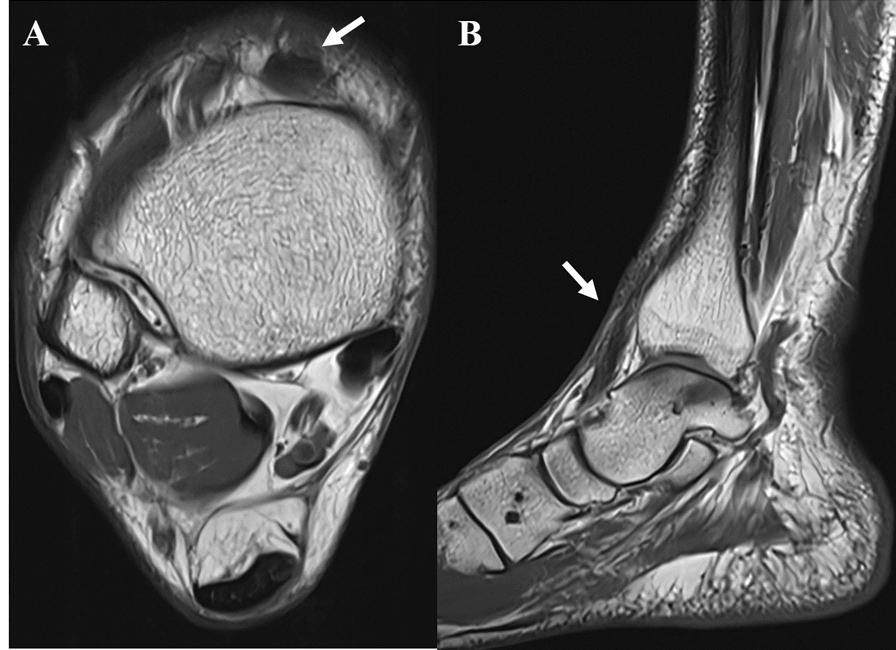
Postoperative magnetic resonance imaging at 1 year. A Axial and B sagittal views demonstrating the continuity of the reconstructed tendon (white arrows). The graft shows low signal intensity and sufficient bulk, indicating favorable remodeling
